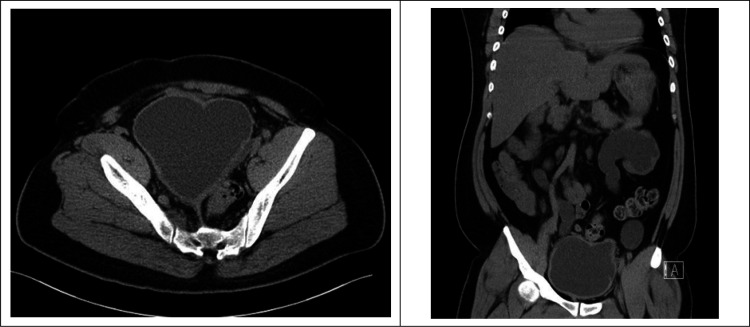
Axial (left) and coronal (right) views of the patient’s abdominopelvic computed tomography scan
